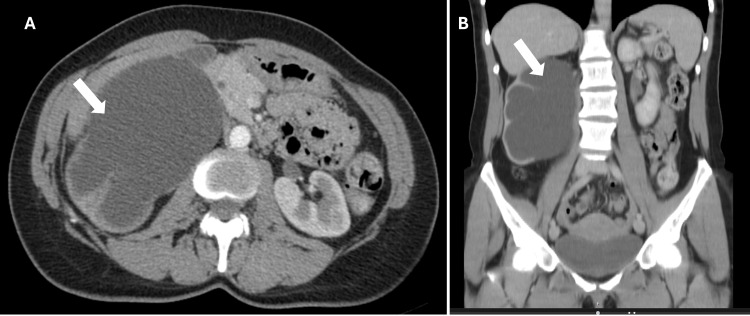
Contrast-enhanced abdominal computed tomography (A) axial and (B) coronal views Marked thinning of the right renal parenchyma and severe dilation of the pelvicalyceal system (white arrows) observed.
